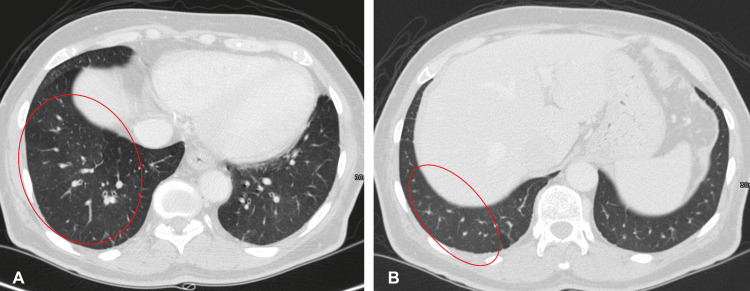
Follow-up computed tomography images (A, B) six months after starting sunitinib; shows complete resolution of lung nodules.
